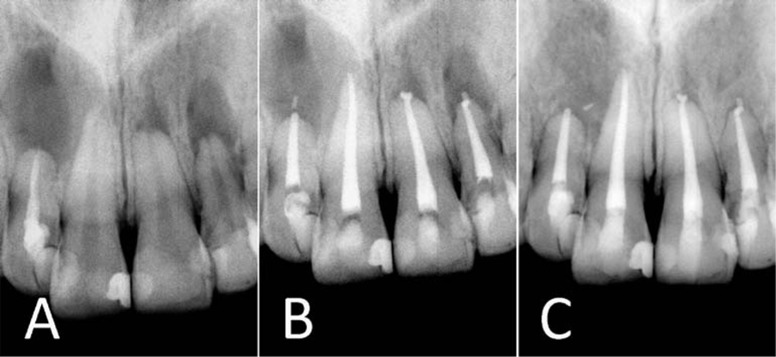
A) Pre-operative radiograph; B) Immediate postoperative radiograph; C) 14-month follow-up radiograph
